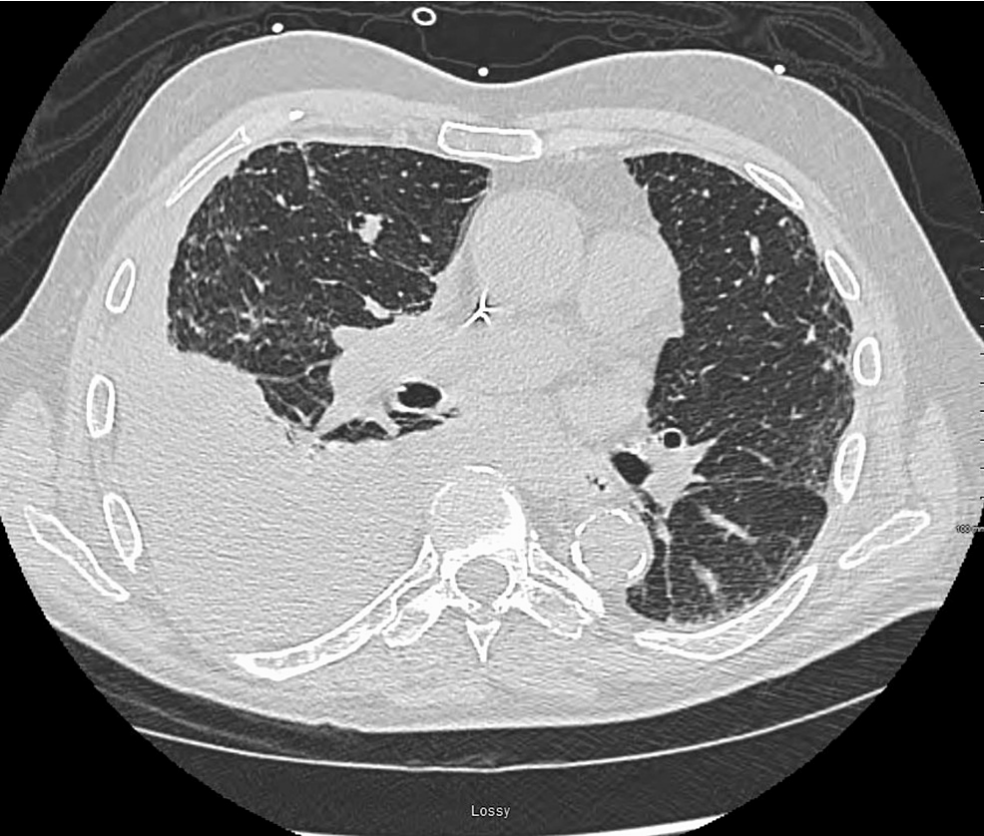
Chest CT (1st Admission)
